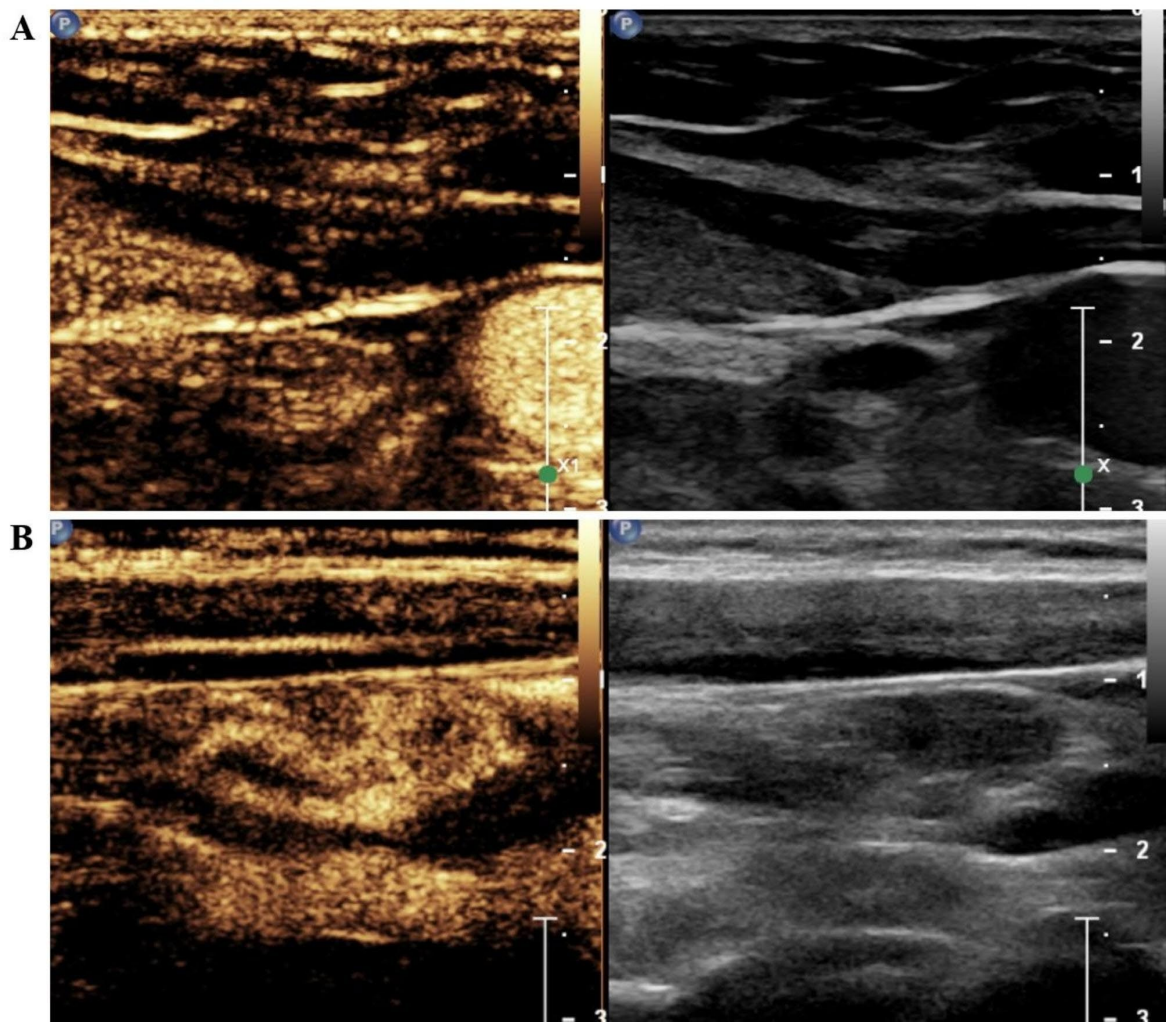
(A) Image of suspicious metastatic lymph nodes reported by contrast-enhanced ultrasound (CEUS) but no metastasis identified by pathological examination. (B) Image of reactive lymph nodes on contrast agent but with tumor metastasis identified by pathological examination
